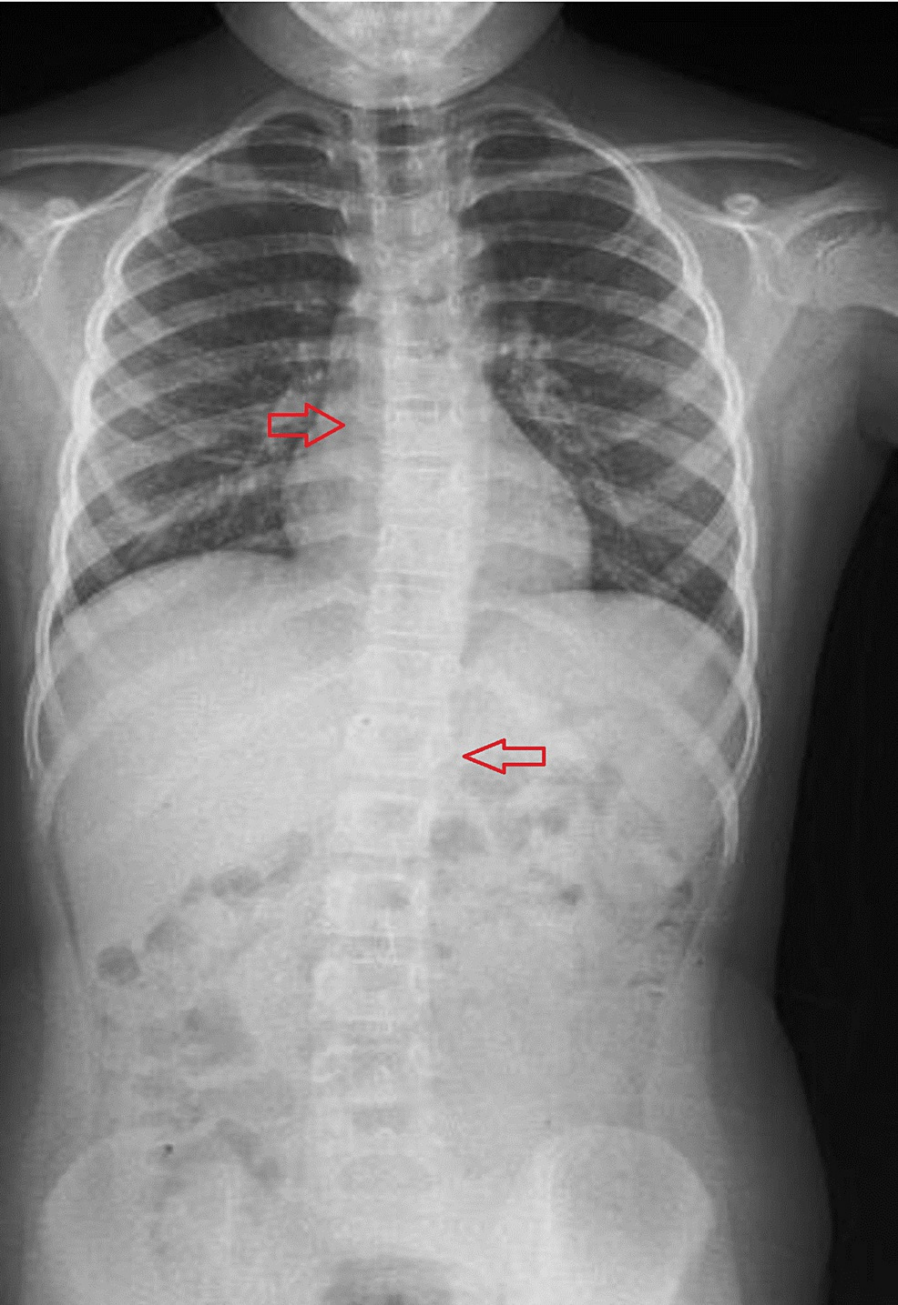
Roentgenography of another patient with early-onset scoliosis (EOS). Left and right arrows indicate the most tilted vertebrae.
